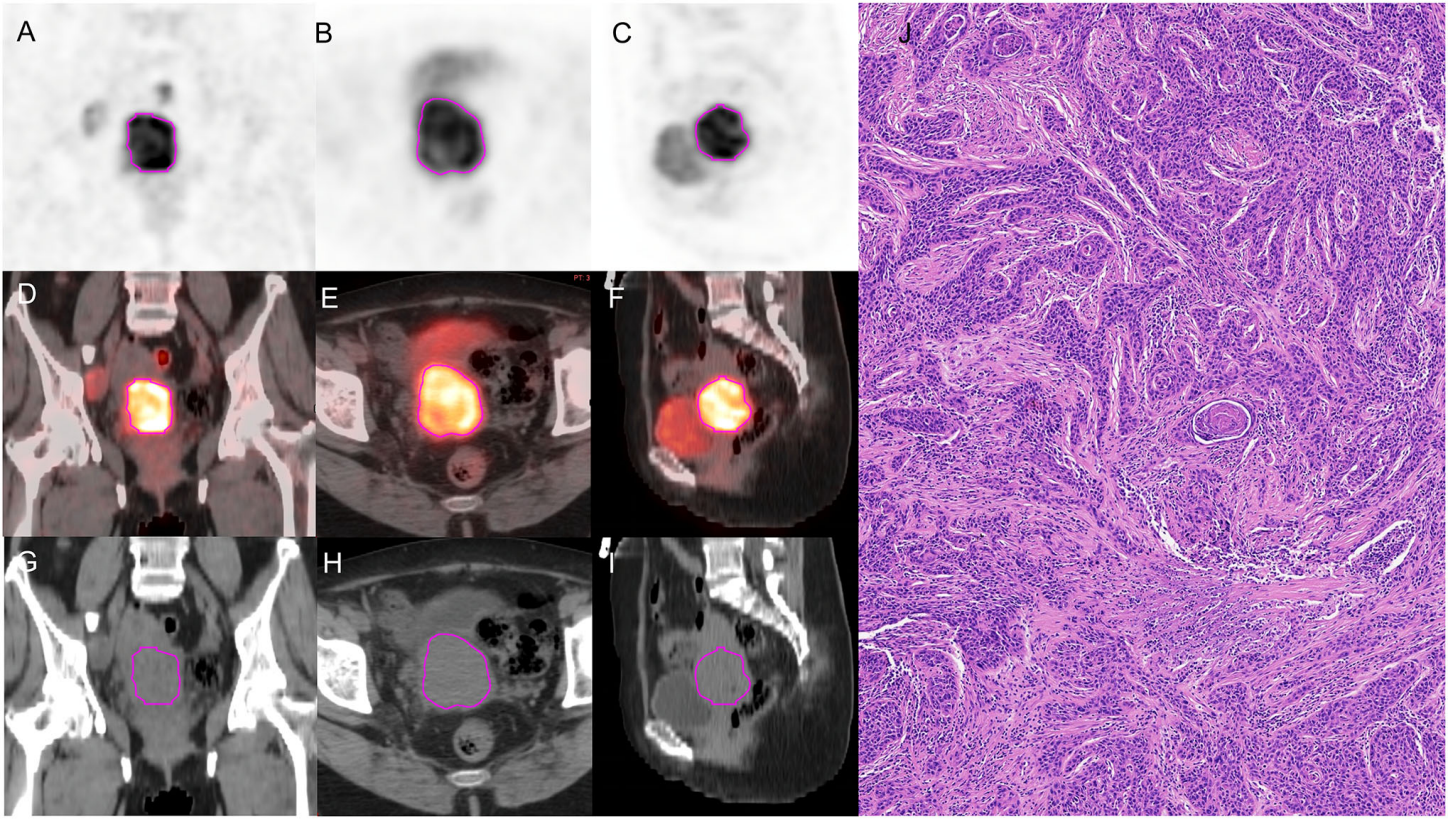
A 53-year-old woman diagnosed with SCC. (A–C) The ROI, labeled in red, was segmented on the coronal, axial, sagittal PET images. (D–F) The ROI, labeled in red, was segmented on the coronal, axial, sagittal fusion images. (G–I) The ROI, labeled in red, was segmented on the coronal, axial, sagittal CT images. (J) pathological examination confirmed SCC.
